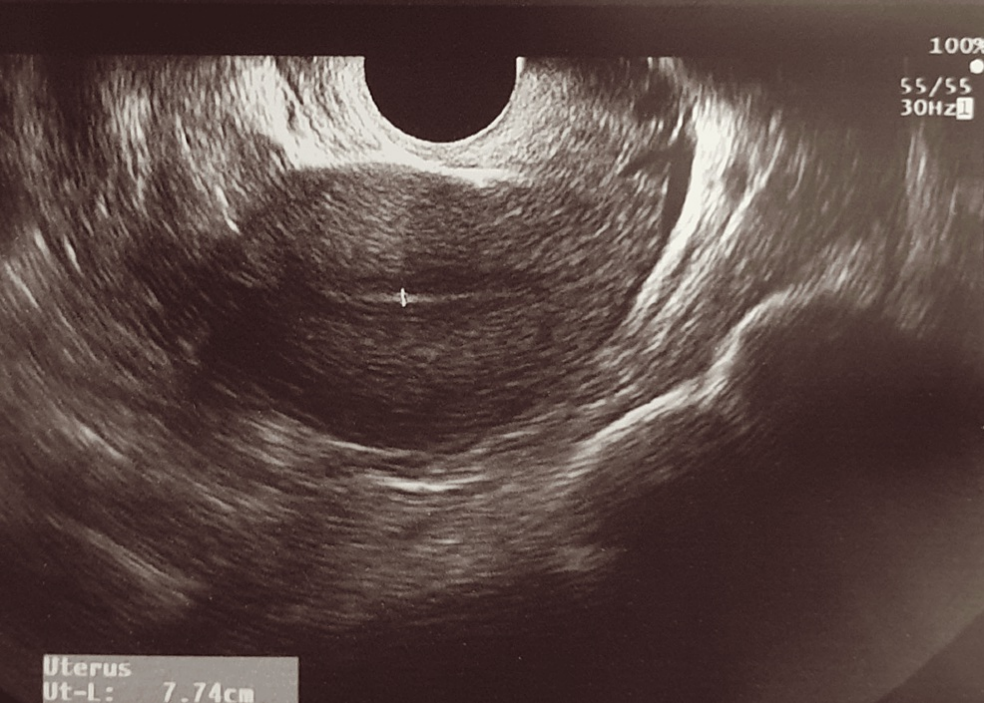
Ultrasound re-examination
